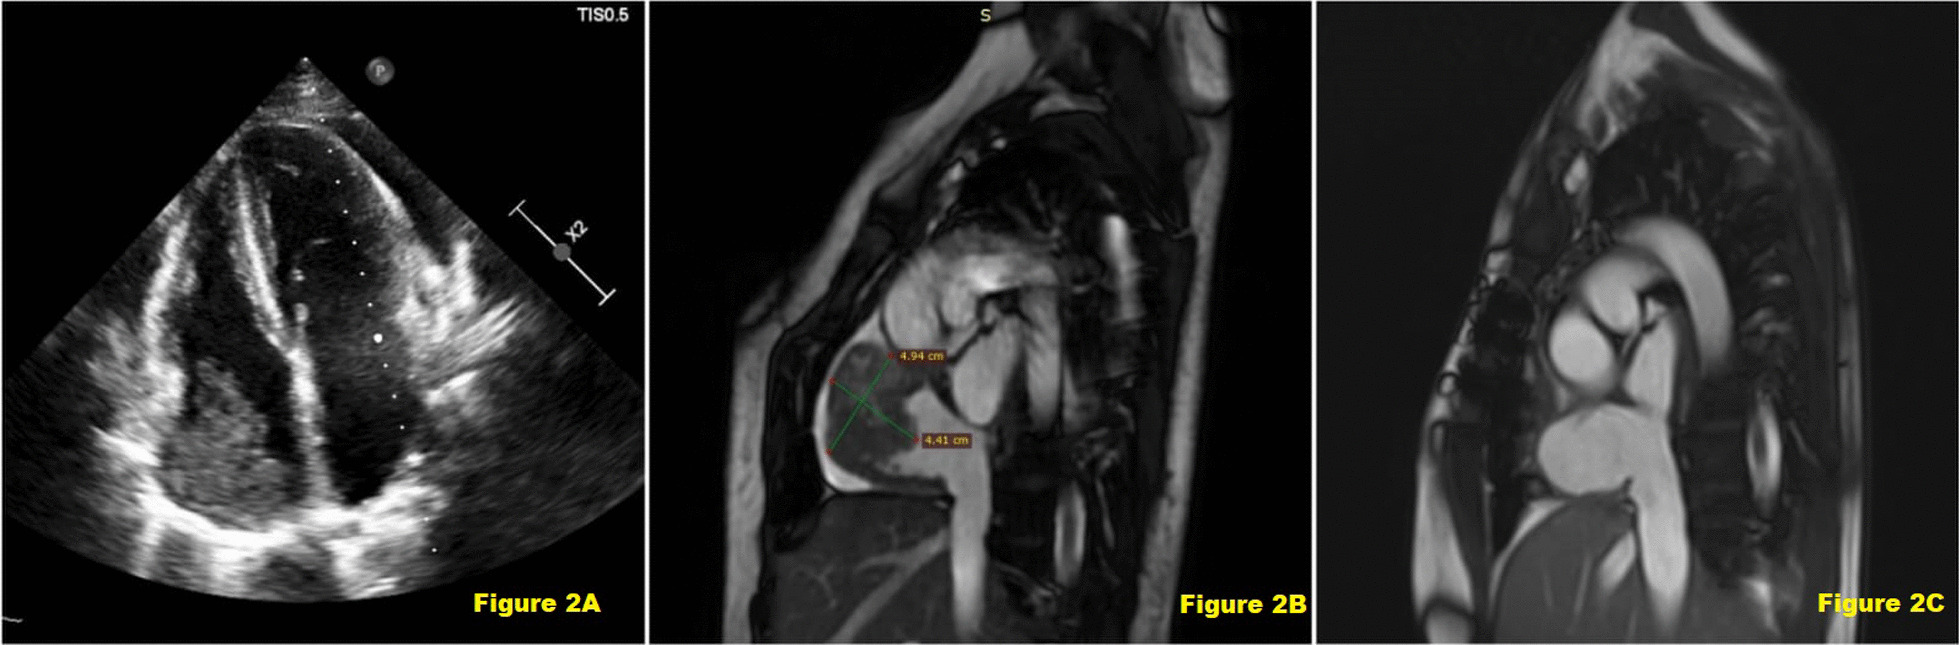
A Preoperative echocardiographic image shows a mass in the right atrium. B Cardiac MRI image that showing an angiosarcoma with a diameter of 4.9 × 4.1 cm (preoperative). C Cardiac MRI image showing Neo-Atrium postoperative 3rd month
